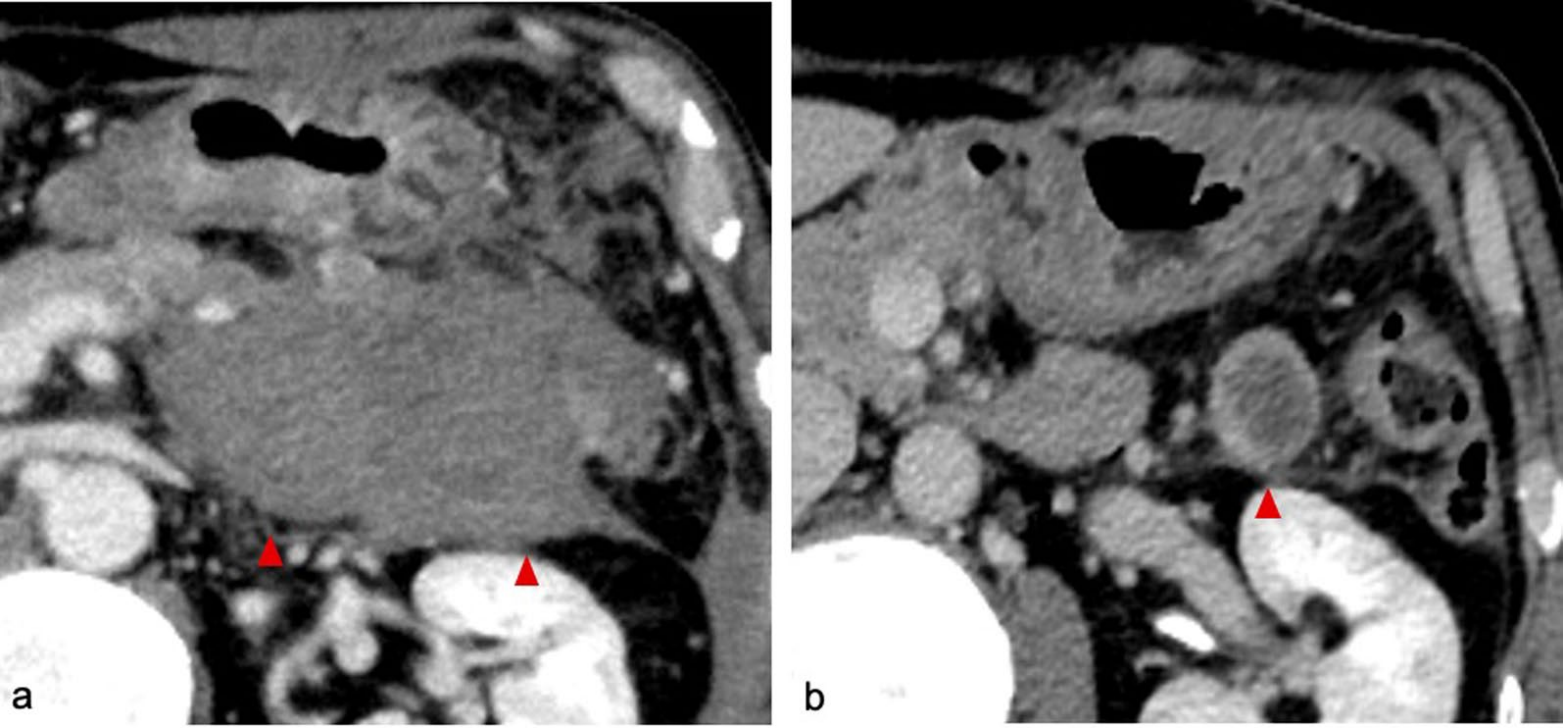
Contrast-enhanced CT of the abdomen. a Contrast-enhanced CT on the fifth hospital day showed a large hematoma (arrowheads) in the left peritoneal space. b Contrast-enhanced CT 5 months after discharge revealed that the hematoma (arrowhead) was remarkably reduced. *CT* computed tomography
